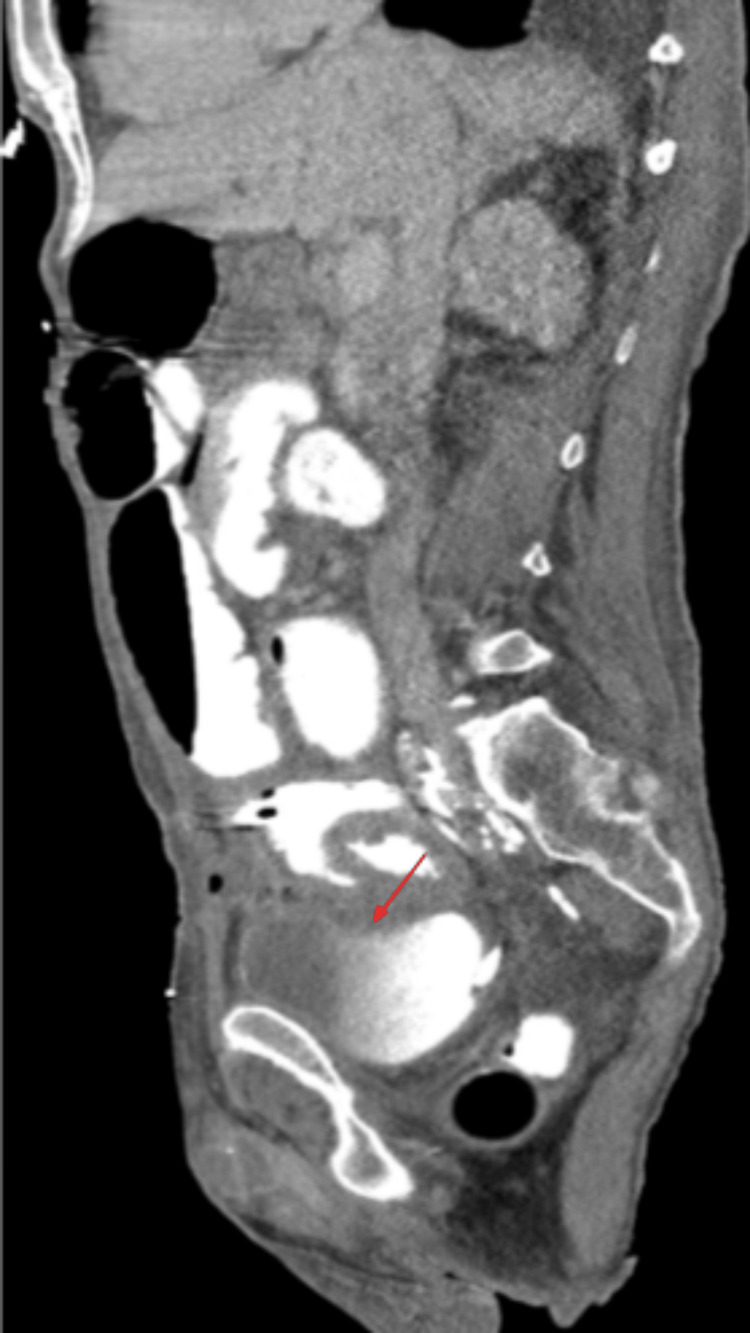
CT abdomen/pelvis in sagittal view demonstrating contrast within the bladder, which represents enterovesicular fistula (red arrow) CT: Computed tomography
